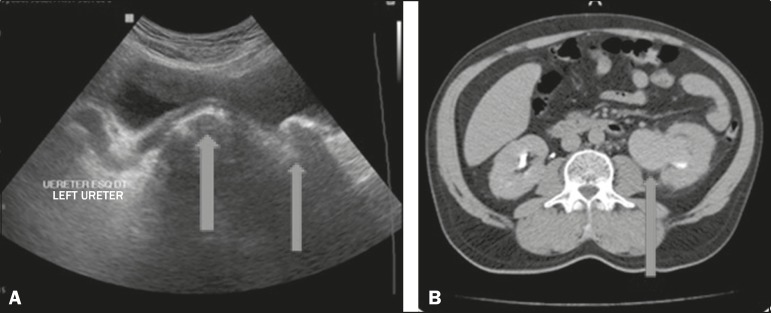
A: Ultrasound showing a calculus within the bladder and another
